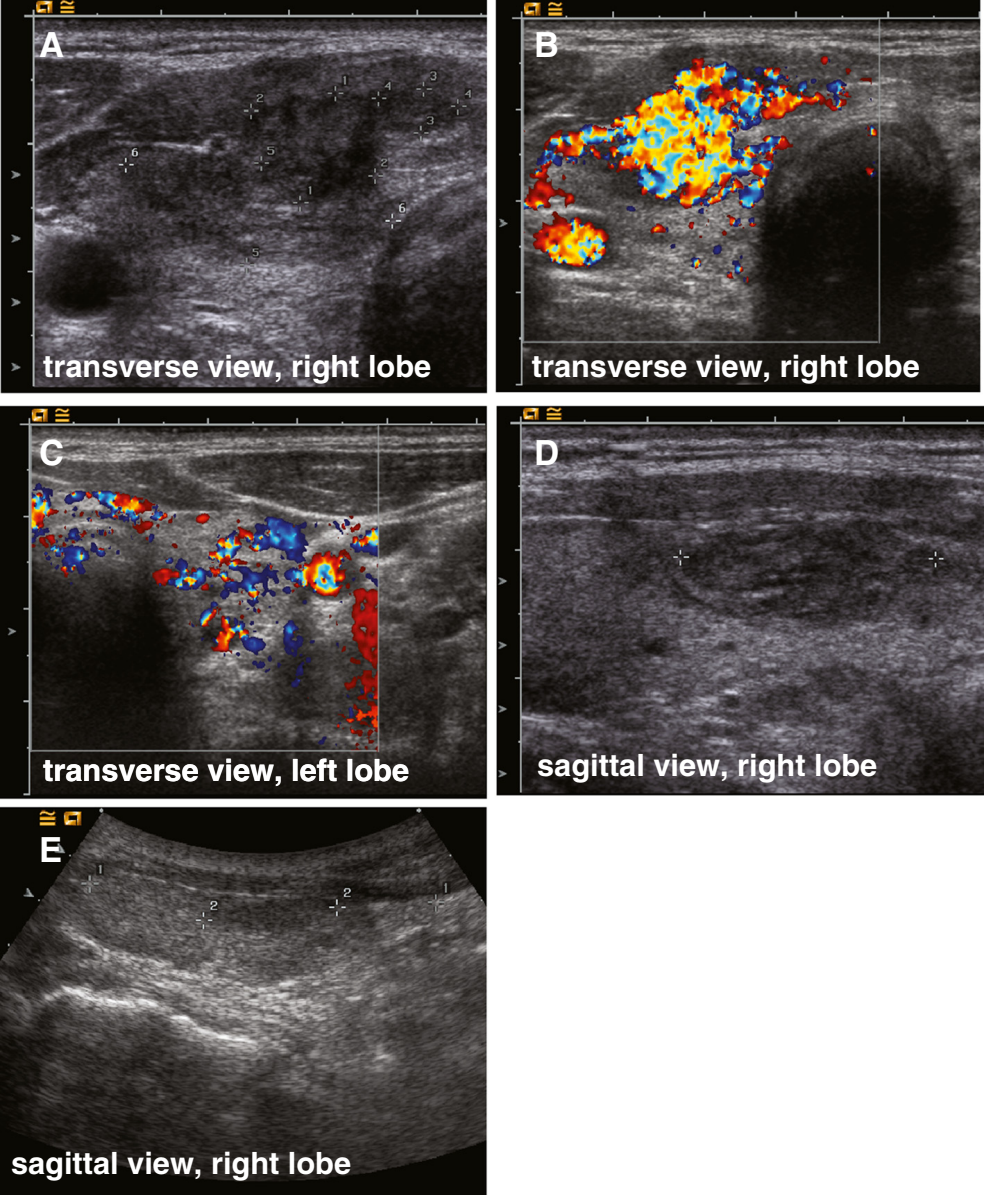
Ultrasound. Thyroid ultrasound transverse (A, B and C) and sagittal view (D and E) of the right and left thyroid lobe showing a diffusely heterogenous gland with a clearly demarcated hypoechoic hypervascularized nodule in an anterior caudal position of the right lobe.
